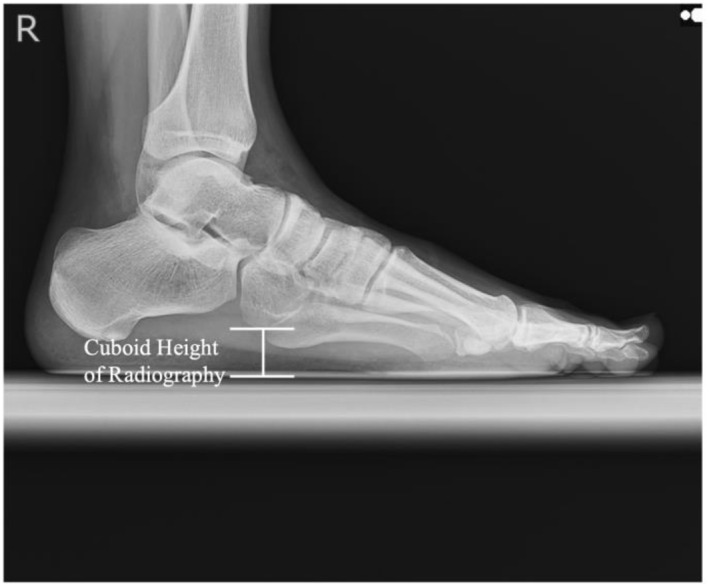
Calculation of the cuboid height using radiography.
